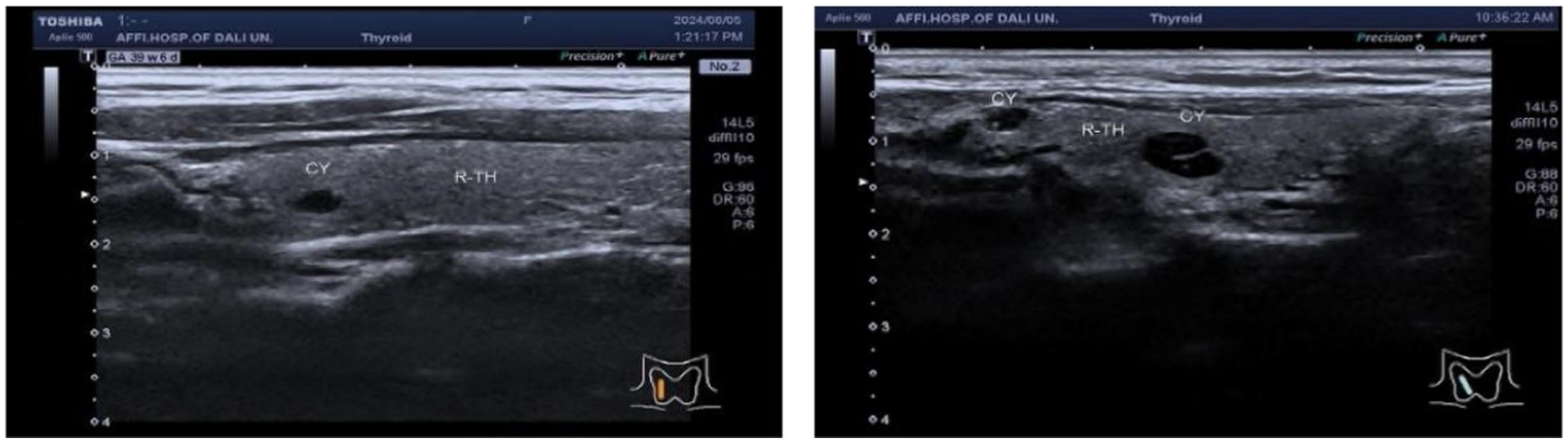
Ultrasound image of cystic nodular lesion in the right lobe of the thyroid gland, C-TIRADS classification: Class 2, multiple cystic nodular lesions in the right lobe of the thyroid gland, C-TIRADS classification: Class 2.
